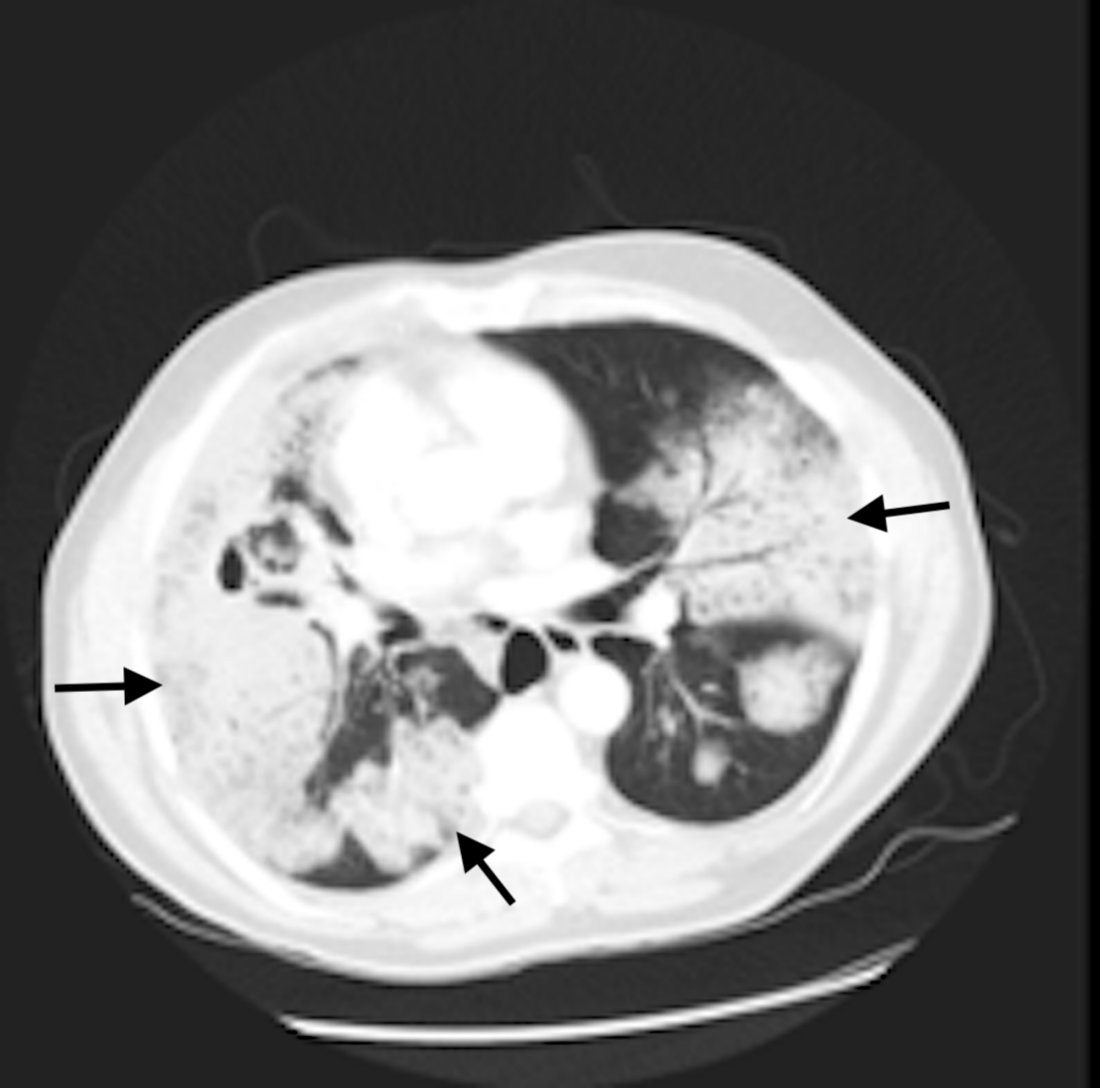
CT Scan 2.5 months prior to diagnosis CT imaging depicts multi-focal consolidation (arrows) with air bronchograms and some alveolar sparing CT: computed tomography
